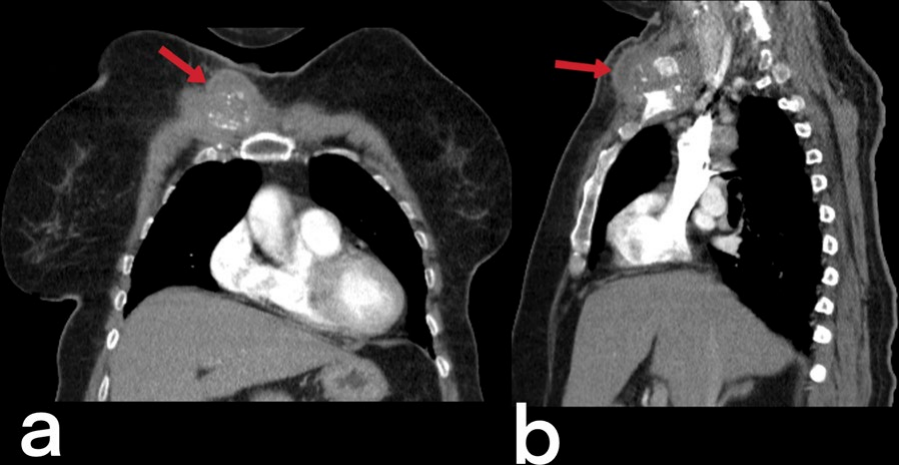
Coronal (a) and sagittal (b) reformatted CT images of a 70-year-old female who has a complaint of swelling in the anterior wall of the chest. Both images reveal mass-like lesions in the right sternoclavicular joint, causing bone destruction containing small calcified areas. The patient was diagnosed with TB after the biopsy
